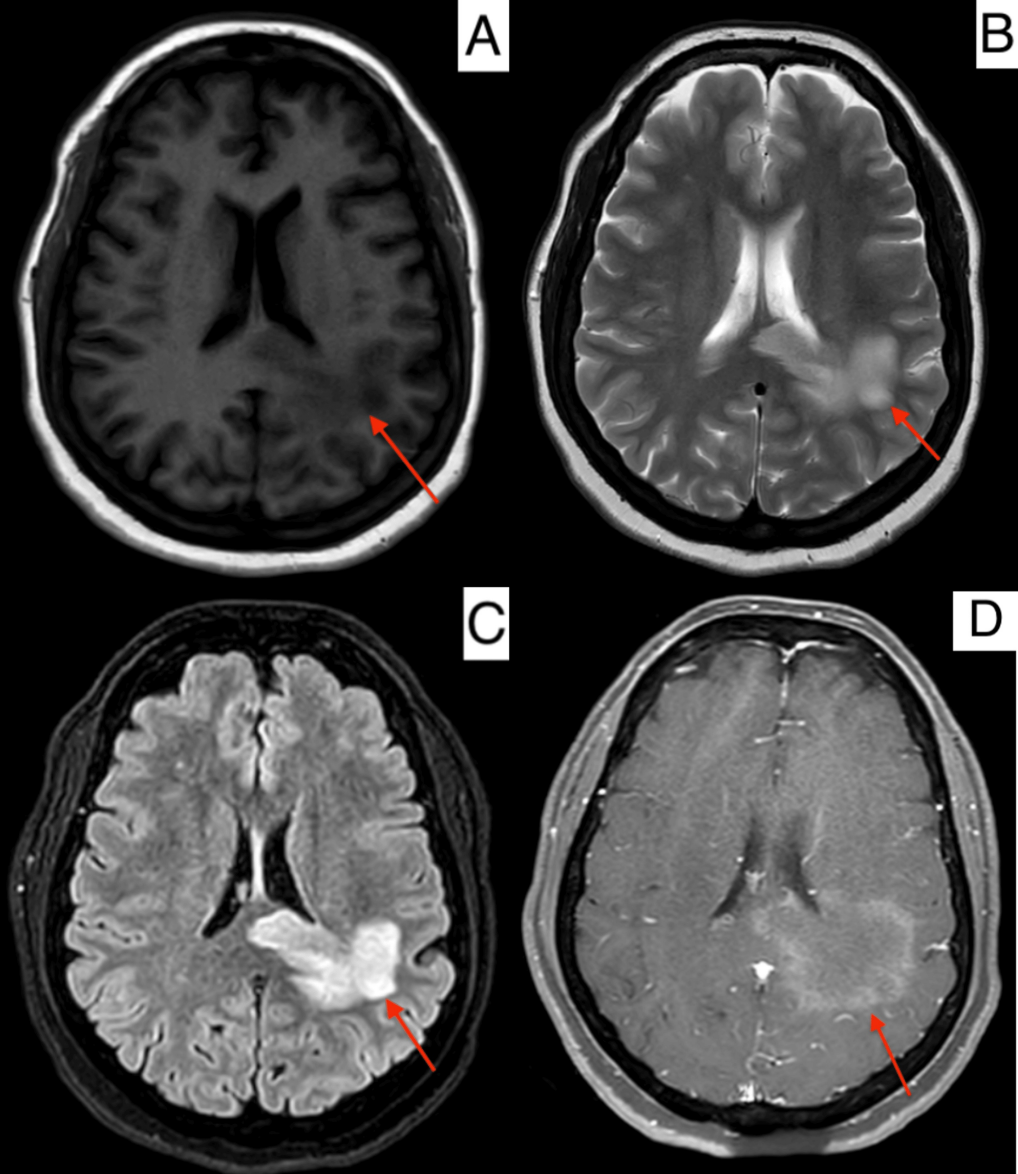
MRI of the brain. T1-weighted image (A), T2-weighted image (B), T2-FLAIR image (C), and T1-weighted contrast-enhanced image (D) showing tumefactive demyelination involving the left parietal region (red arrow) June 1, 2024 MRI: magnetic resonance imaging, FLAIR: fluid-attenuated inversion recovery
